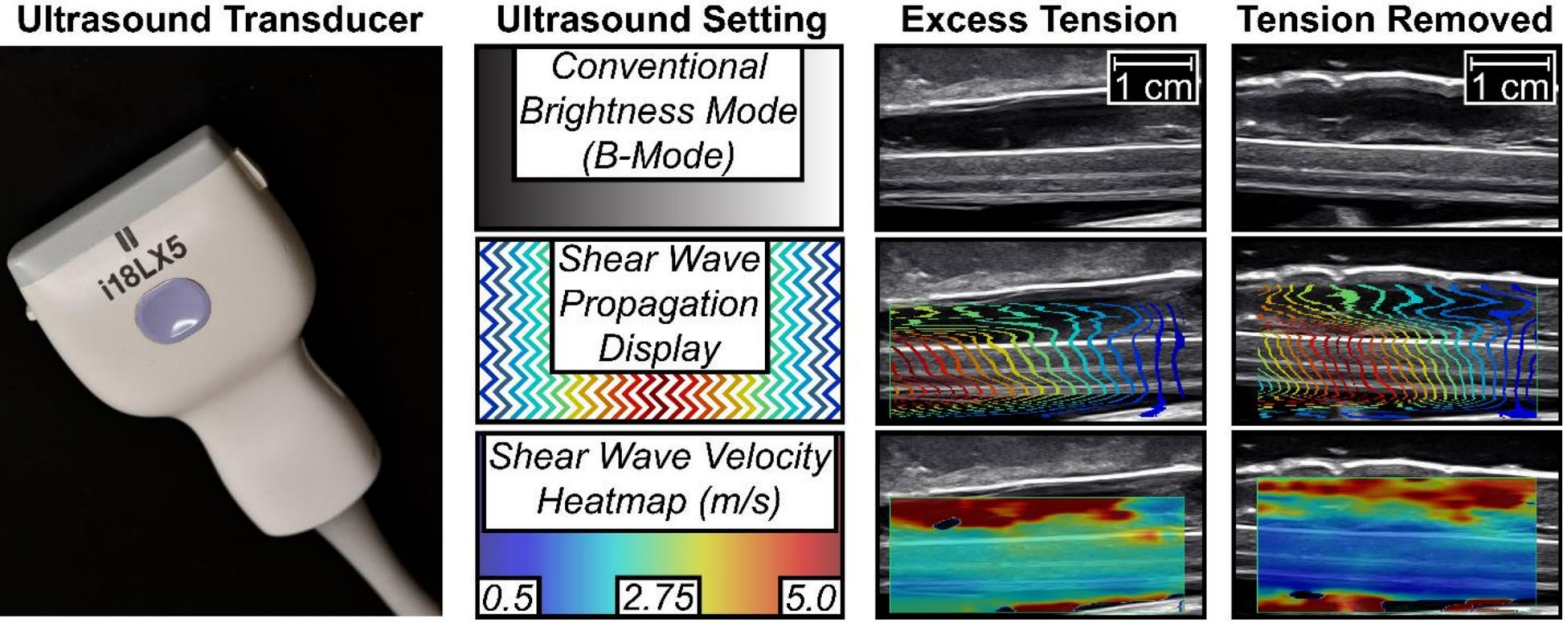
The promising utility of elastography to intraoperatively differentiate the physical state of a patient’s spinal cord is shown. Here, a posterior vertebral column subtraction osteotomy (PVCSO) was performed to treat tethered cord syndrome (TCS) by alleviating excess tension across the spinal cord. In this case, an i18LX5 ultrasound transducer attached to an Aplio i800 (Canon Medical Systems, Otawara, Japan) was used to capture the data. This figure is adapted from Kerensky et al. (2024), "Tethered spinal cord tension assessed via ultrasound elastography in computational and intraoperative human studies," Commun Med, CC BY 4.0 (https://doi.org/10.1038/s43856-023-00430-6). Components from Fig. 4 in Kerensky et al. (2024) have been re-labeled and repositioned with the addition of a supplemental image of the transducer for succinctness and clarity. (See: https://creativecommons.org/licenses/by/4.)
